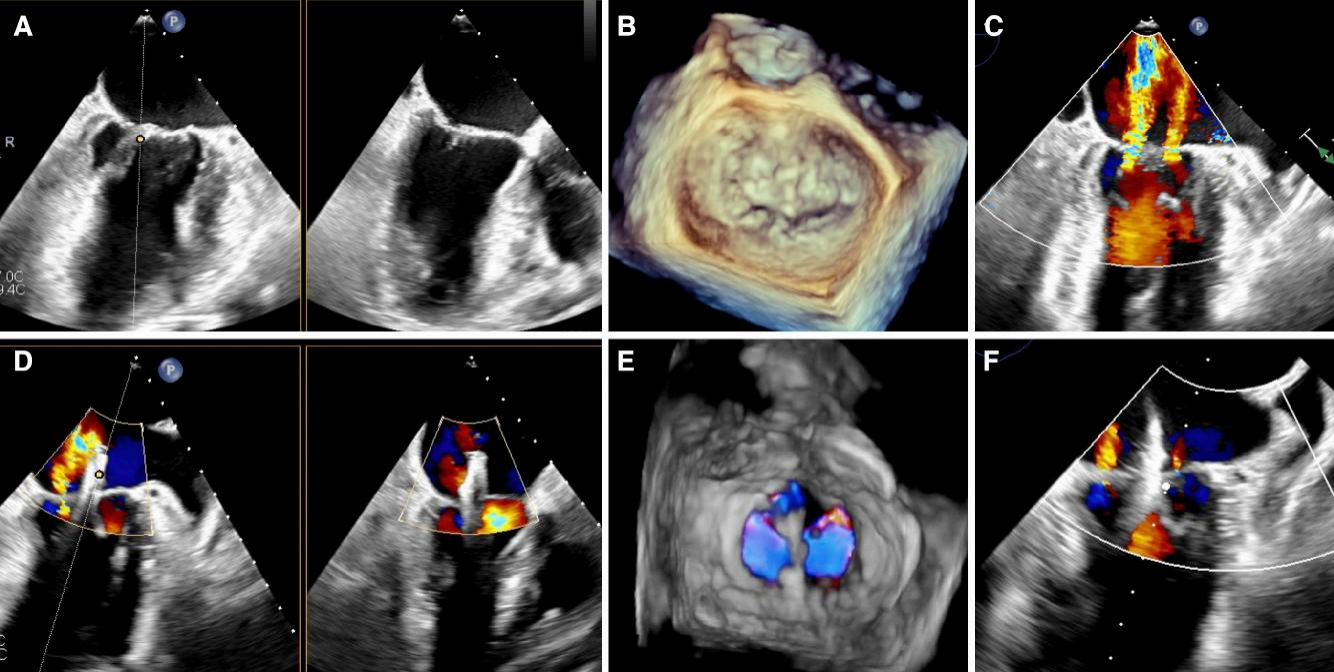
A 77-year-old female with a 14-year history of atrial fibrillation was re-hospitalized for acute decompensated heart failure. Transoesophageal echocardiography (TEE) showed moderate-to-severe (3+) mitral regurgitation (MR) with reduced and flattened leaflet coaptation (*Panels A and B*; Supplementary material online, *Video S1*), suggesting atrial functional MR (AFMR). Mitral regurgitation originated from A1P1 (vena contracta = 4 mm) and A3P3 (vena contracta = 5 mm) segments (effective regurgitant orifice area = 0.38 cm^2^, regurgitant volume = 55 mL), separated by a 6 mm regurgitant-free region at A2P2 (*Panel C*; Supplementary material online, *Video S2*). Posterior leaflet length was 8.5 mm at A2P2 but <7 mm at the regurgitant segments. The mitral valve area was 4.7 cm^2^.
